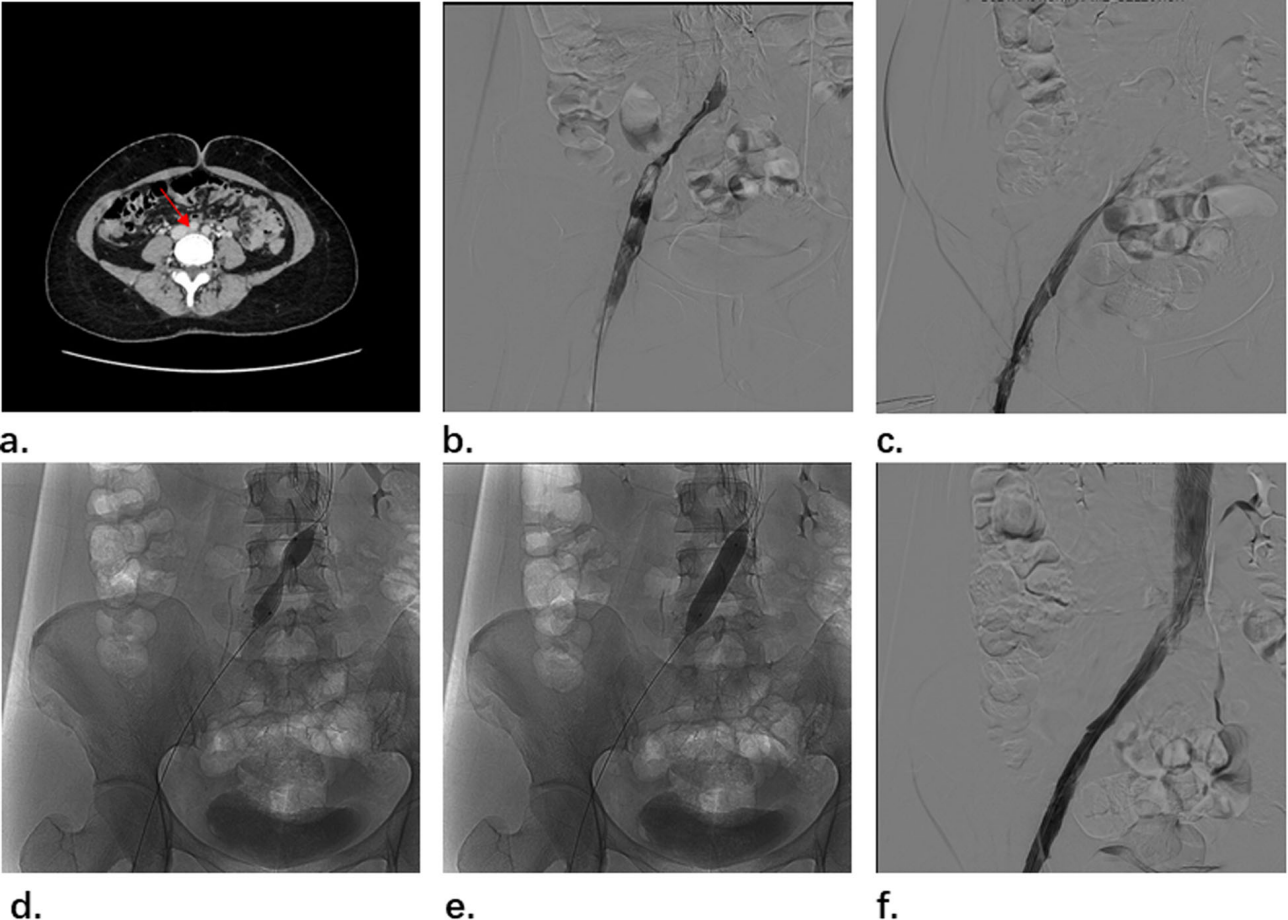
a CT showed May-Thurner syndrome. b venography showed that significant stenosis in left common iliac vein. c Three days later, the venography showed that the thrombus was almost completely dissolved. d-e 12 mm*40 mm PTA balloon dilatation of left common iliac vein. f left common iliac vein stenosis relieved and the inferior vena cava was visible
